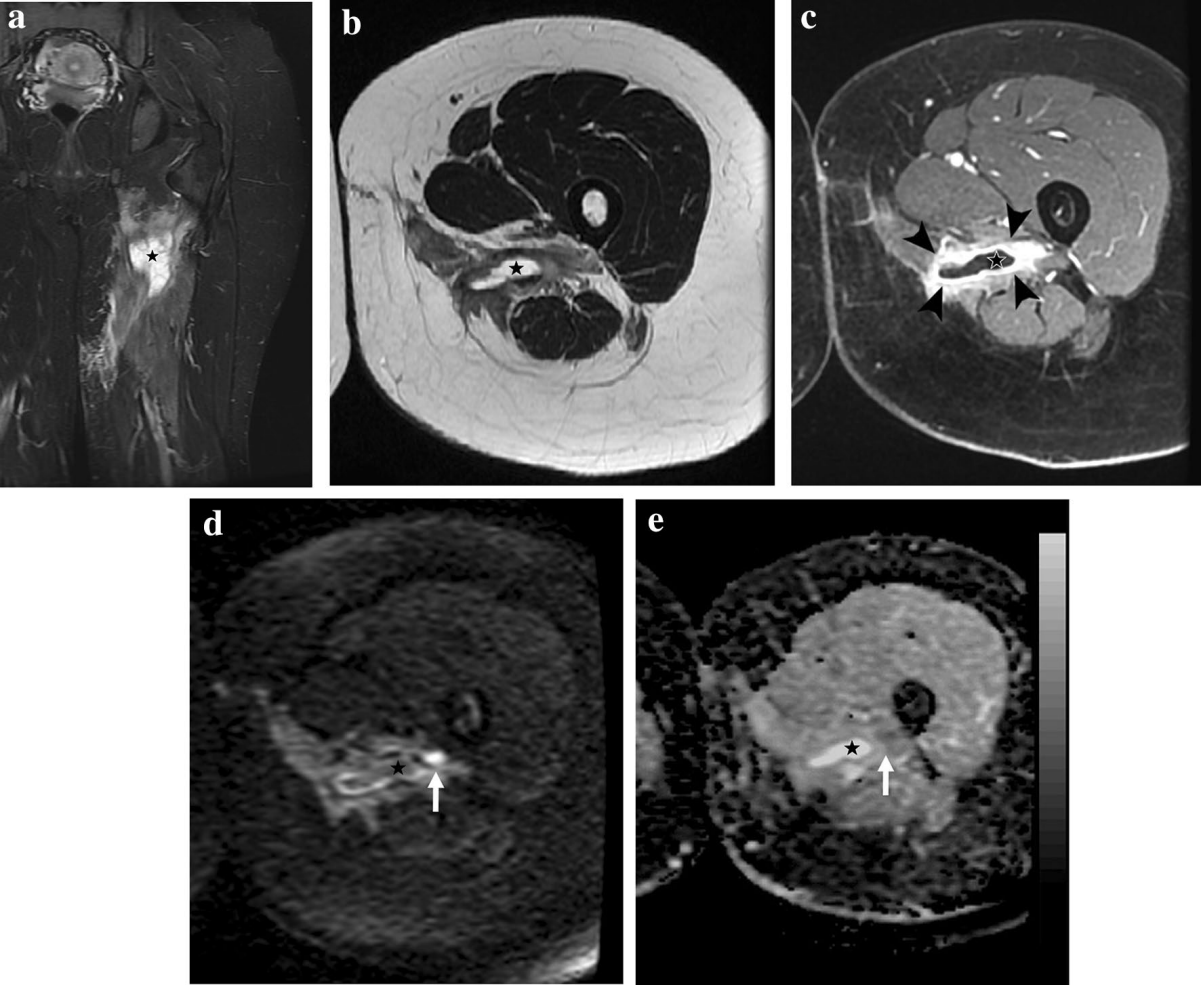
Example of the confounding aspect of the post-surgical changes on all the sequences except for *b* = 1000 DWI. Twenty-nine-year-old woman with residual spindle cell sarcoma in the proximal left thigh after surgical excision with positive margins. a Coronal STIR image showing diffuse soft tissue post-surgical edema with a large deep fluid collection (*star*). b Axial T2w and c axial post-contrast fat-sat T1w GRE images show thick and irregular enhancing tissue (*arrowheads*) at the periphery of the fluid collection (star). d Axial DWI at the same level reveals a small hyperintense nodule suggestive of residual disease. e The corresponding axial ADC map shows a barely visible focal hypointense lesion with an ADC value of 1.29 × 10^–9^ m^2^/s (ADC value of the scar 1.71 × 10^–9^ m.^2^/s)
